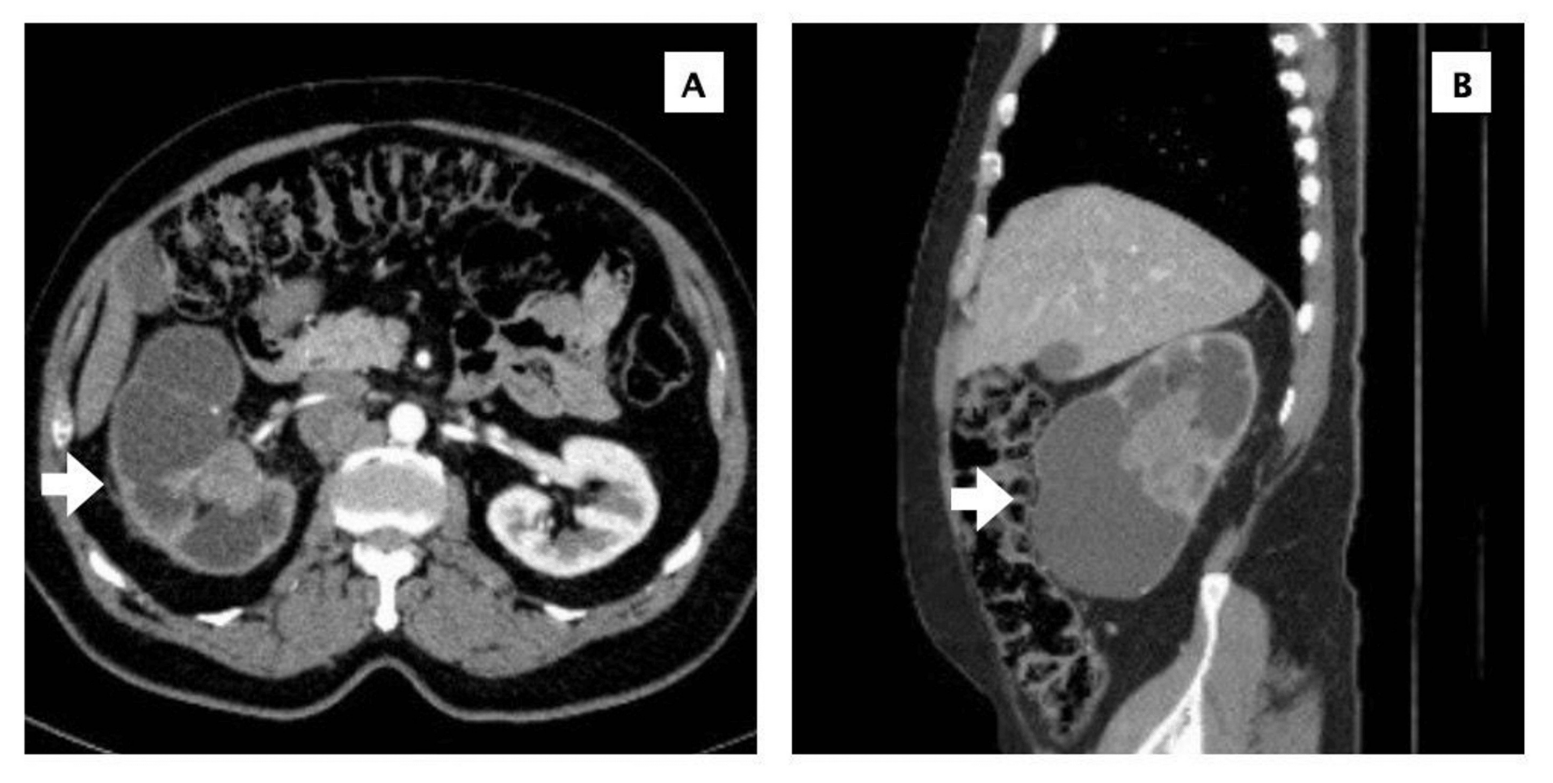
Abdominal CT, transverse view (A) and sagittal view (B), showing large right renal mass (white arrow) replacing the renal pelvis.
